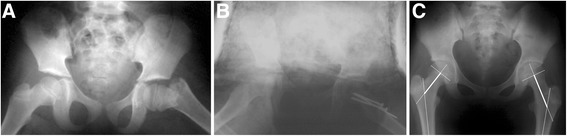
Representative radiographs of the femoral neck overgrowth after a type II femoral neck fracture. a A patient with a displaced type II fracture of the left femoral neck. b The two cannulated screws and one smooth k-wire did not pass through the proximal femoral growth plate after closed reduction and internal fixation. c Overgrowth of the femoral neck (8.5 mm) was visible 8 years following injury
